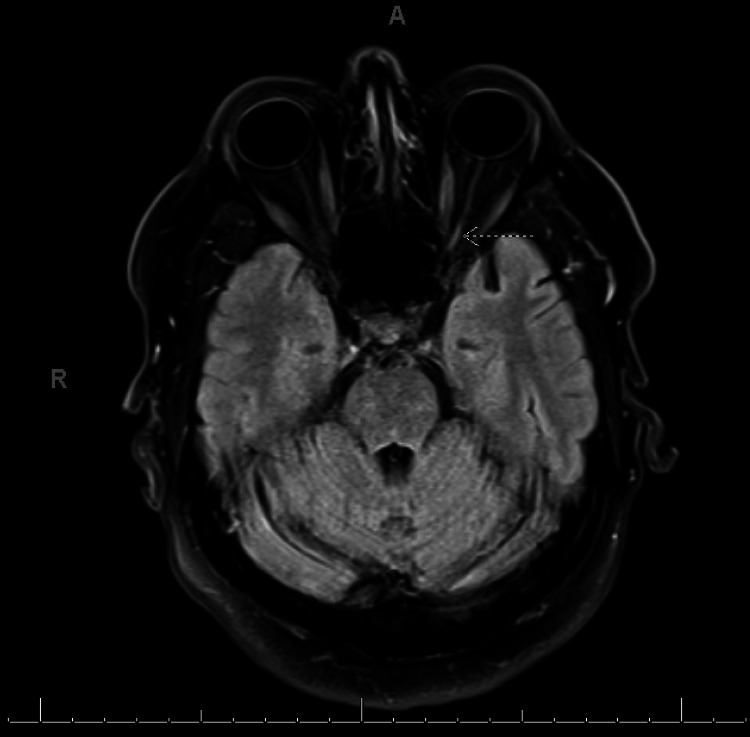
MRI orbit axial T2-weighted with fluid attenuated inversion recovery. Shows left greater than right optic nerve atrophy with nonspecific, high signal in the posterior intraorbital segment and canicular segment of the left optic nerve (white, dotted arrow).
